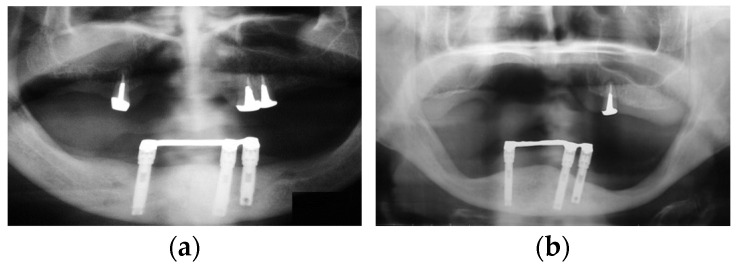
Bone resorption in a patient with implant-supported overdenture: (a) a panoramic X-ray image at the delivery of overdenture; (b) a panoramic X-ray image at 19-year follow-up. Source: the authors.
